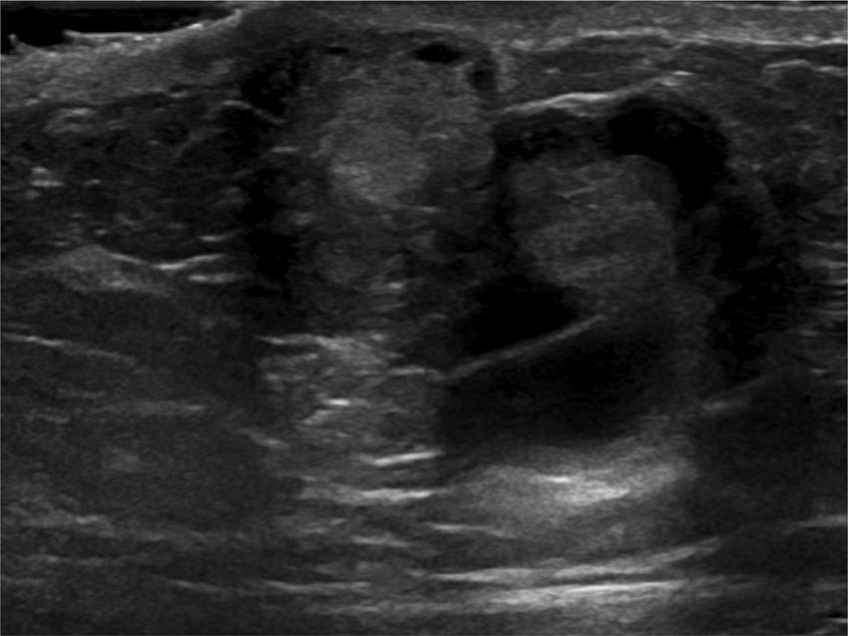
Ultrasound of retroareolar area of palpable concern – second view.
